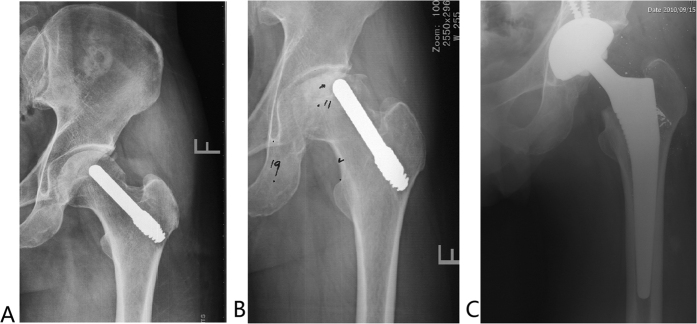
Anteroposterior radiograph of a 49-year-old man with Association Research Circulation Osseous (ARCO) stage II osteonecrosis of the left hip undergoing a tantalum porous implantation was taken intraoperatively (A) and 14 months postoperatively (B), at which time the collapse had progressed and the joint space had narrowed. The patient underwent total hip arthroplasty 14 months after tantalum porous implantation (C).
